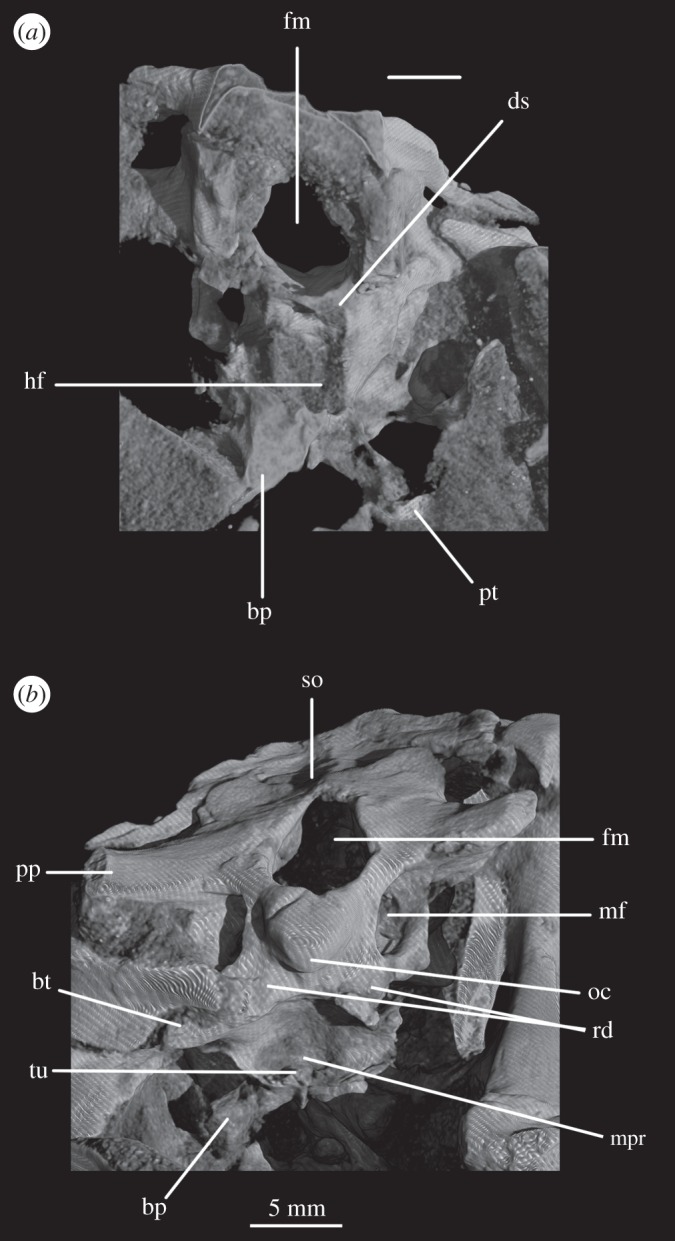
CT reconstructions of braincase of SAM-PK-5867 in (*a*) anterior view in cross section through skull and (*b*) posteroventral view. For abbreviations, see table 1.
